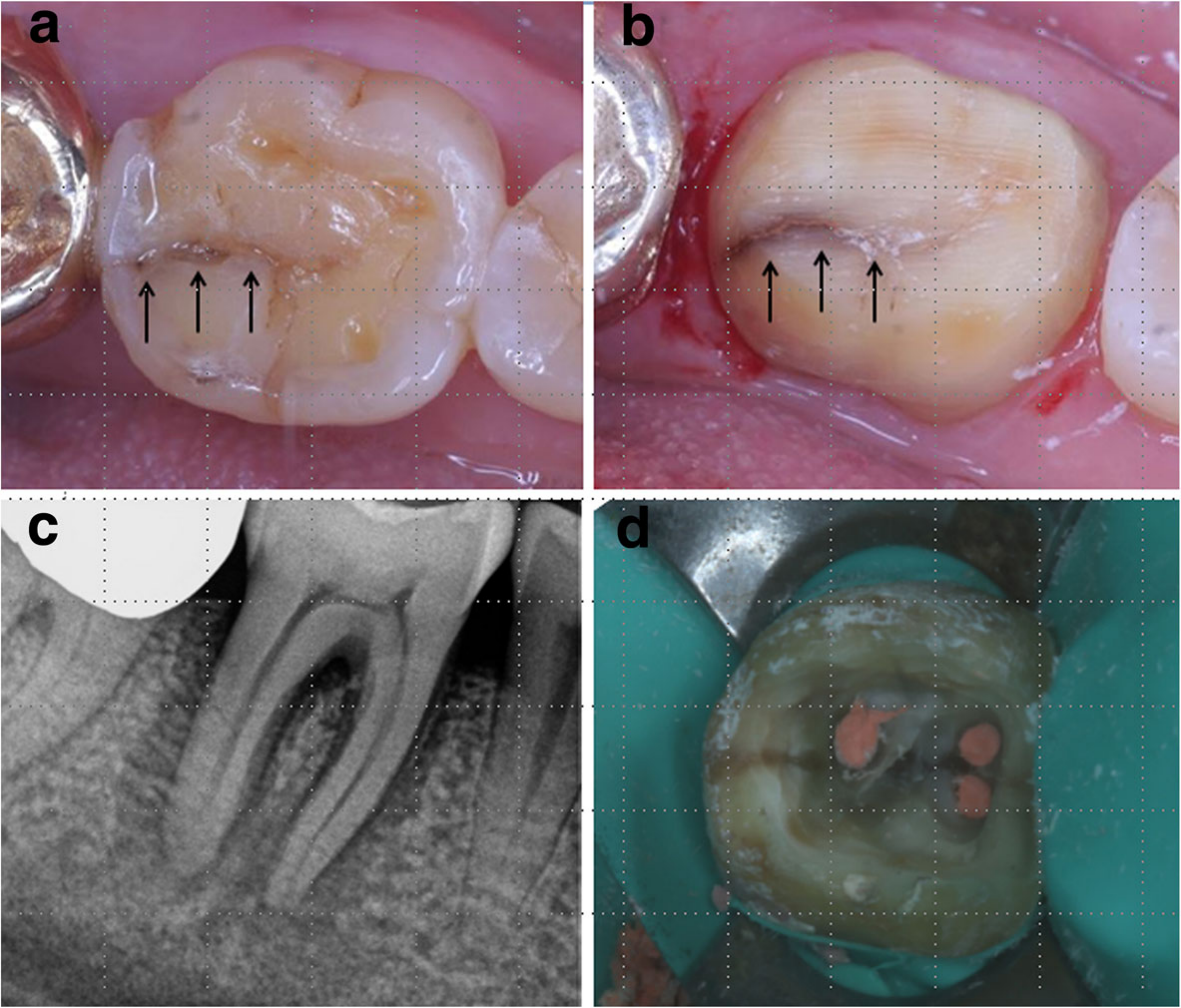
Crack that developed in a mandibular first molar with no restoration. a, b A distal crack line (black arrows) was observed in the central groove of the mandibular first molar. c Periapical lesion was detected in the periapical radiograph. d During an endodontic procedure, a crack line extending to the pulp chamber floor was seen
